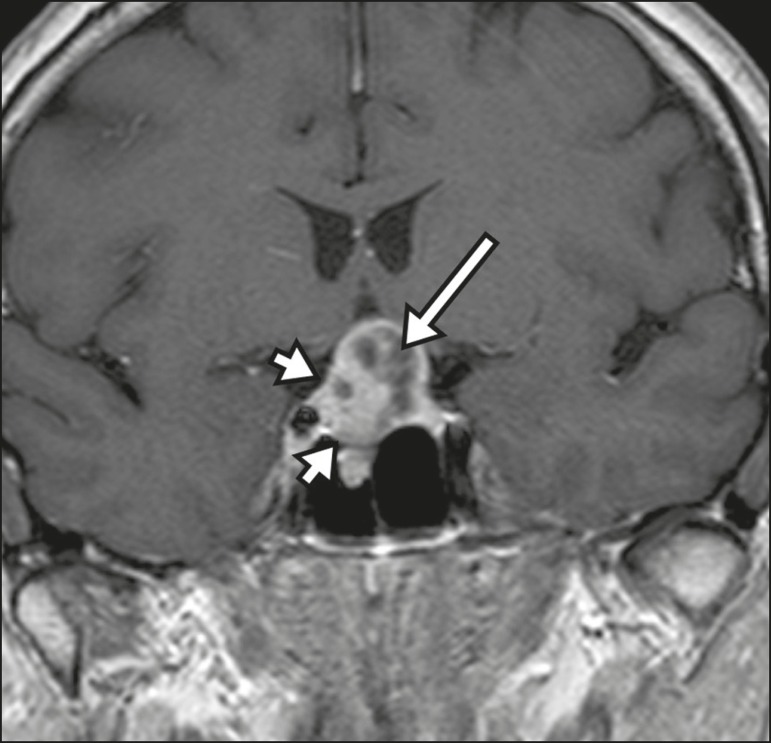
Adamantinomatous craniopharyngioma. Contrast-enhanced MRI scan showing a
